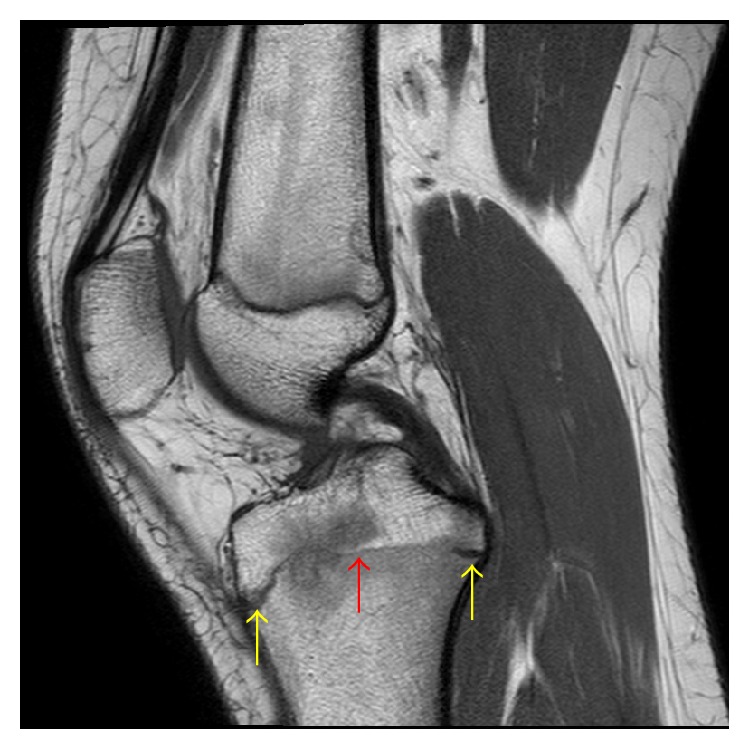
A 14-year-2-month-old female with FOPE zone of the proximal tibia. Findings: Sagittal T1 weighted image shows partial closure of the proximal tibia physis centrally (red arrow) with the physis still open along the periphery of the tibia (yellow arrows). The distal femur physis is narrowed but still open by comparison. Low T1 signal intensity corresponding to the periphyseal edema is again seen spanning the proximal tibia physis. Technique: sagittal T1 MRI (TR = 621.991, TE = 15), ST = 3 mm, spacing = 3.3 mm, FOV = 14 cm, and matrix = 340 × 277.
